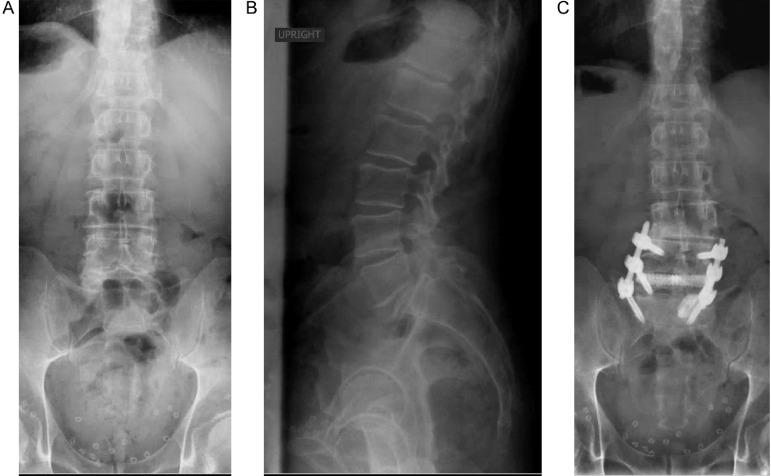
A: pre-operative AP x-ray of the lumbosacral spine demonstrating multilevel spondylolisthesis and degenerative disease. B: pre-operative lateral x-ray of the lumbosacral spine demonstrating multilevel spondylolisthesis and bilateral pars defect at L5-S1. C: post-operative AP x-ray of thoracolumbar spine film demonstrating placement of TLIF cage and pedicle screws. D: post-operative lateral x-ray of thoracolumbar spine with interval placement of TLIF cage and modest correction of spondylolisthesis.
